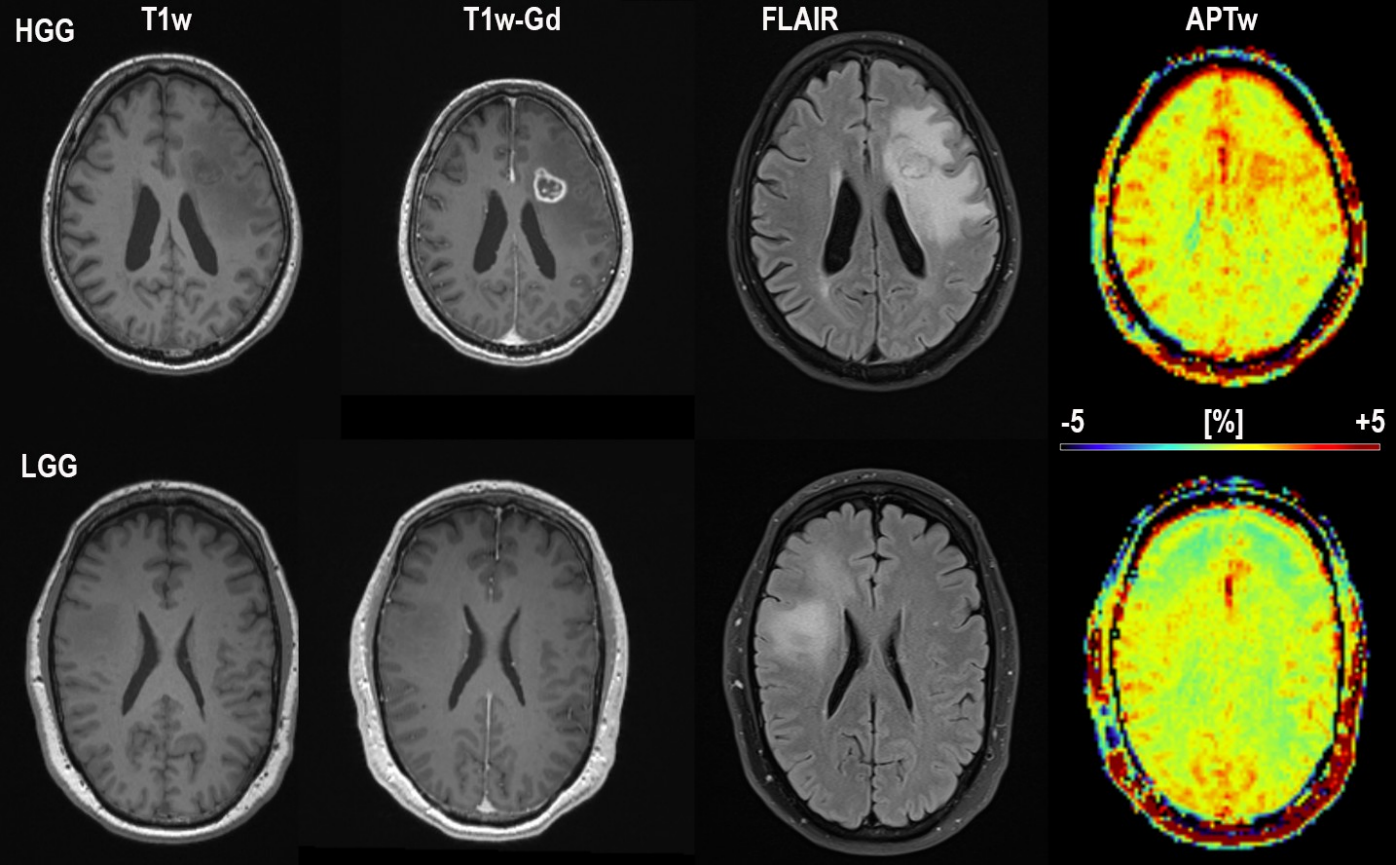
Conventional MRI; T1-MPRAGE without contrast, T1-MPRAGE with Gadolinium, FLAIR and APTw in color for subject one (HGG; top) and subject 3 (LGG; bottom) The APTw signal intensity for the high grade glioma subject at the top is distinctly higher than for the low grade glioma subject at the bottom. Also notice the increased APTw signal in blood vessel regions. Subject 1 HGG (misdiagnosed by reader 1 and 3 as MET, correctly diagnosed by APTw) and subject 3 LGG (correctly diagnosed by all)”.
